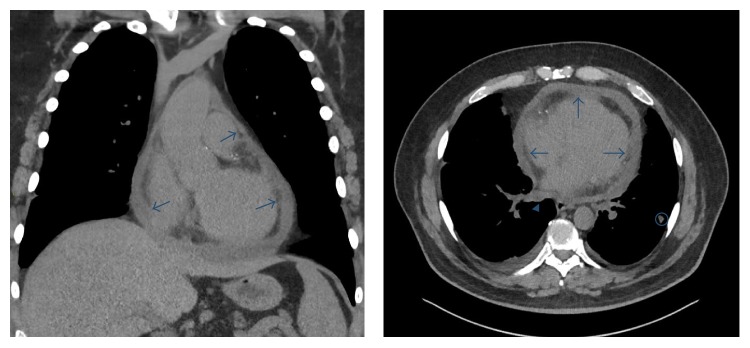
Chest CT imaging. Coronal view of noncontrast chest CT scan showing hyperattenuated pericardium (arrows). Axial chest CT scan showing the same diffusely thickened pericardium. Enlarged mediastinal lymph node (arrowhead). Note the solitary nodule in the Left lower lobe (encircled).
